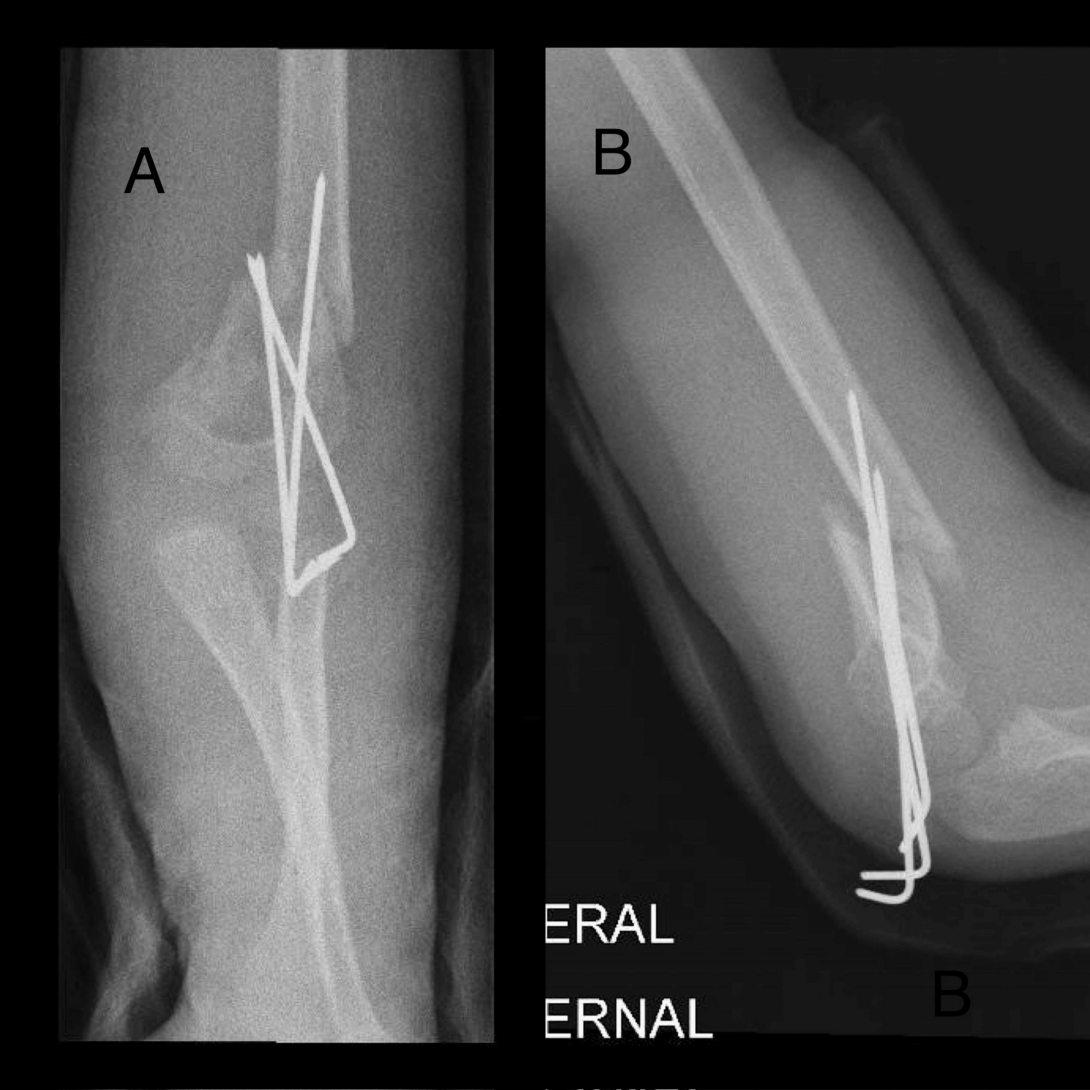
X-ray upon referral to our clinic (day 5 post initial CRPP) (A) anteroposterior view; (B) lateral view CRPP: closed reduction percutaneous pinning
